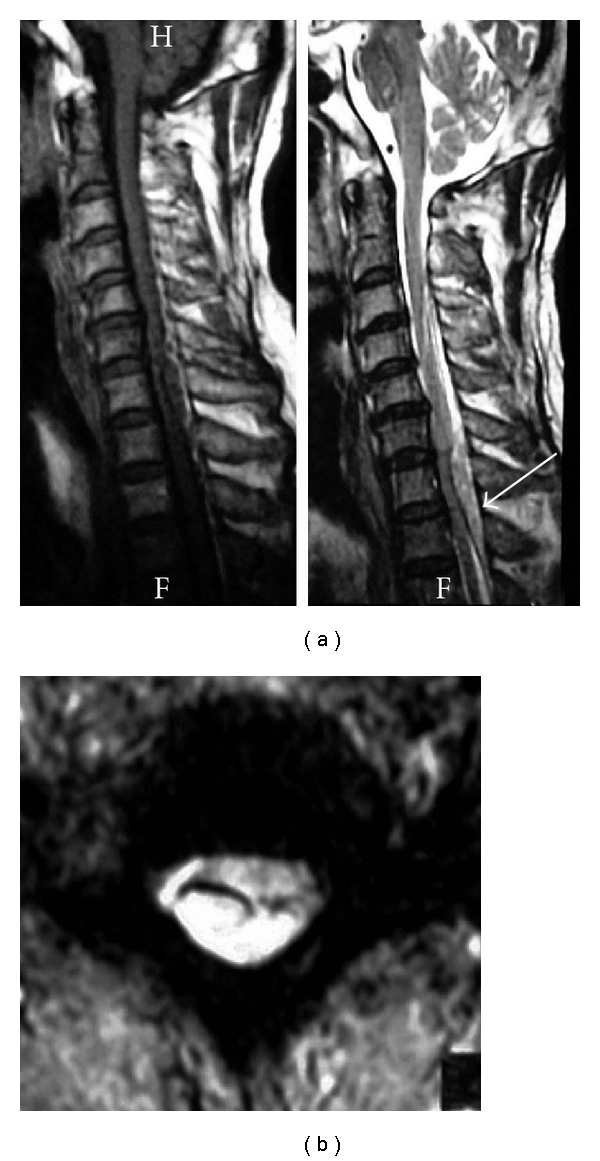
Preoperative sagittal (a) and axial (b) MR images showing a right posterolateral dominant epidural hematoma at the C6-T1 level with spinal cord compression arrow.
